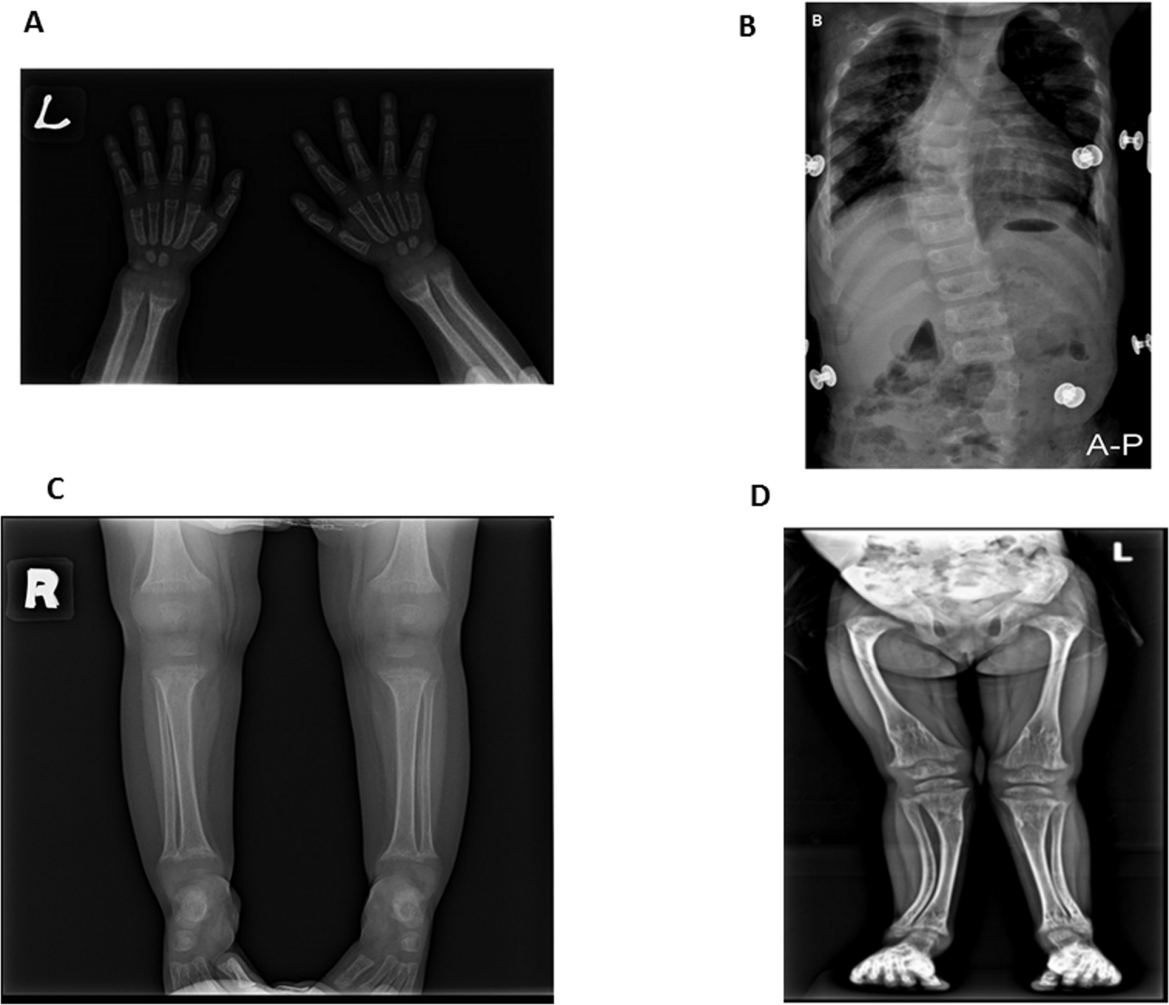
(A) Antero-posterior radiograph of the patient’s hand with rickets demonstrates cupping and fraying of the metaphyseal region. (B) Antero-posterior radiograph of the patient’s chest showing scoliosis of the thoracolumbar spine with Cobb’s angle of 31degrees. (C) Antero-posterior radiograph of lower extremities revealed widening between the distance of the end shaft and epiphyses. (D) The curvatures of legs revealed genu valgum with intermalleolar distance (IMD) of 5.0cm.
